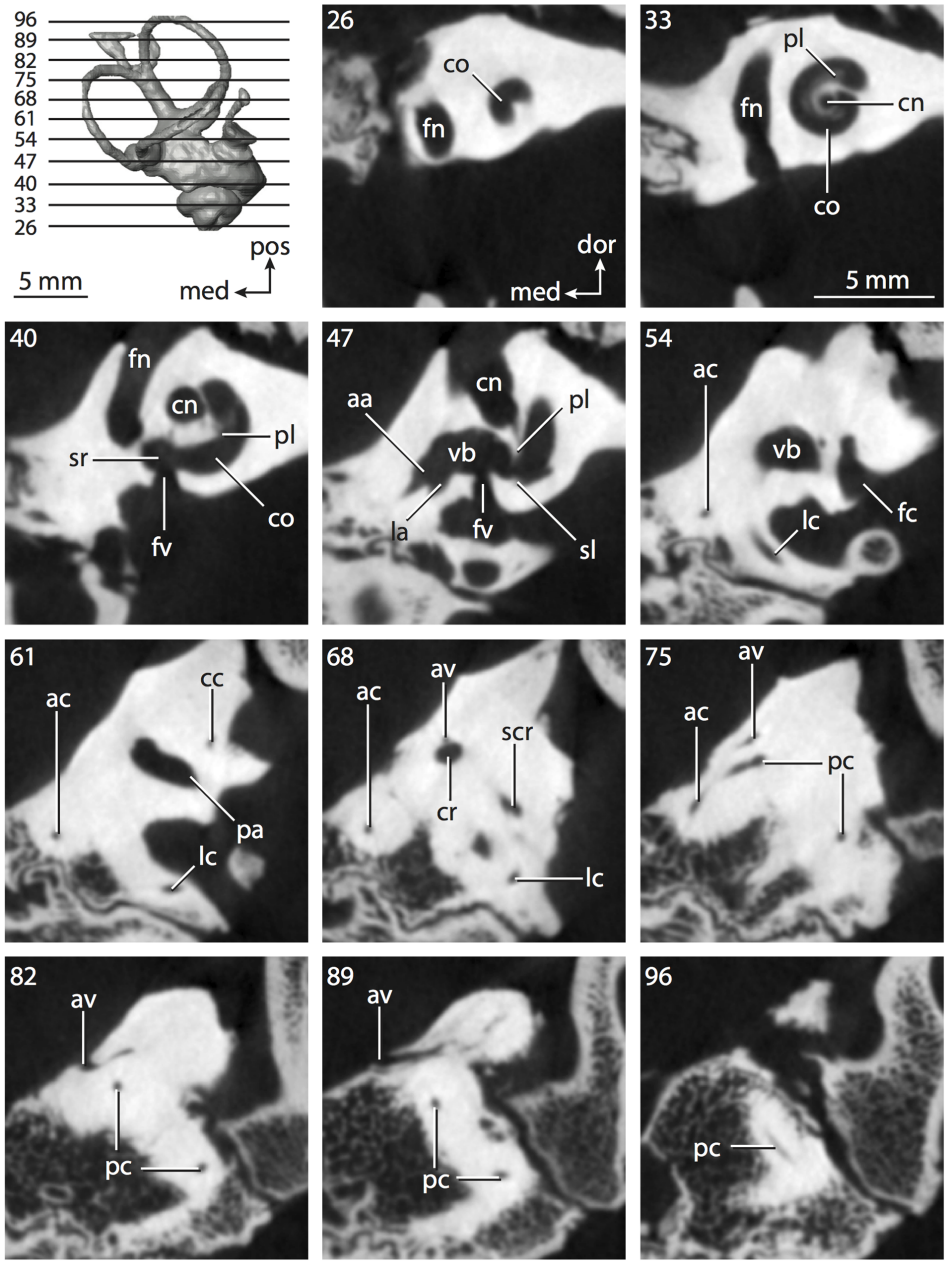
CT slices through ear region of*Orycteropus afer*. Abbreviations listed at the end of the Materials and Methods section.
